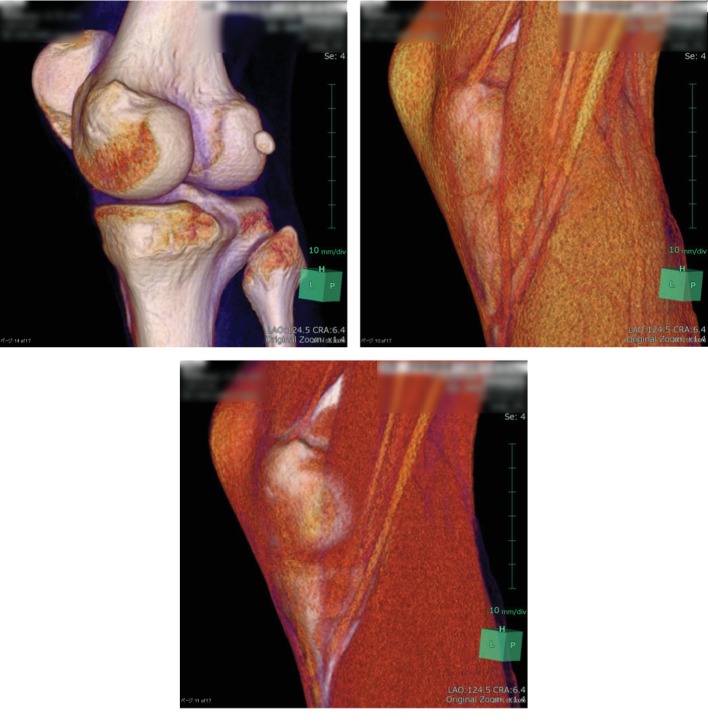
Three-dimensional reconstructed computed tomography images. There was no anatomical abnormalities in the bone and pes anserinus.
